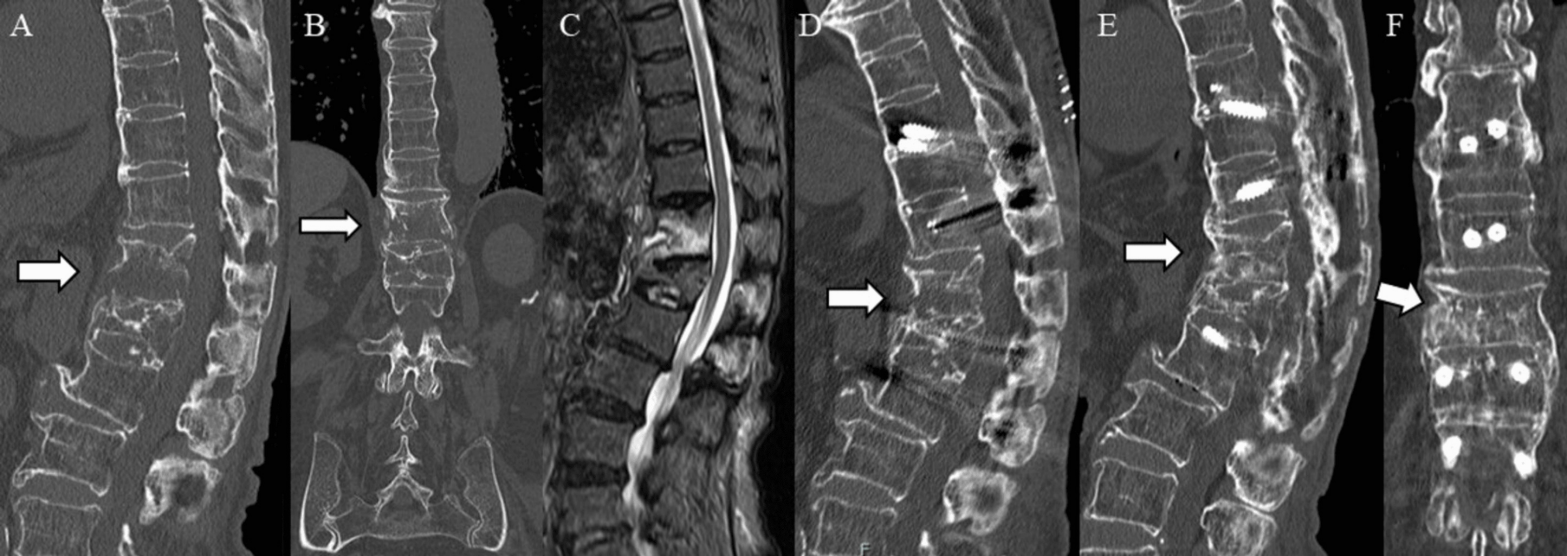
Pre- and postoperative images A. Preoperative plain computed tomography in the sagittal section (local kyphosis angle, 17°; anterior wall height ratio, 135%); B. Preoperative plain computed tomography in the coronal section; C. Preoperative magnetic resonance imaging (MRI) in the sagittal section; D. Postoperative plain computed tomography in the sagittal section (local kyphosis angle, 28°; postoperative anterior wall height ratio, 101%); E. Plain CT sagittal section at one year postoperatively. Good bone fusion; F. Plain CT coronal section at one year postoperatively. Good bone fusion.
